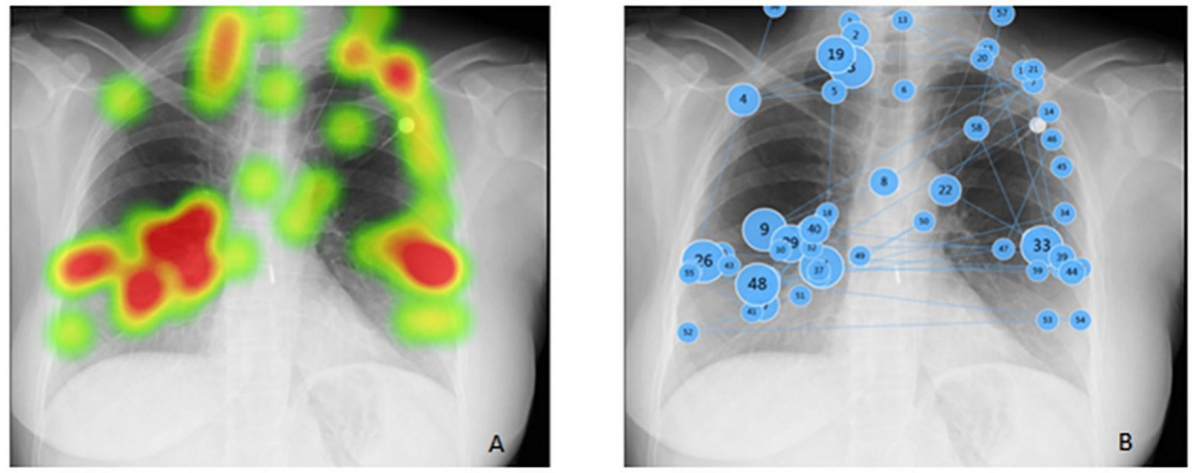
The time spent and location of the gaze by the medical student looking at chest x-ray.
